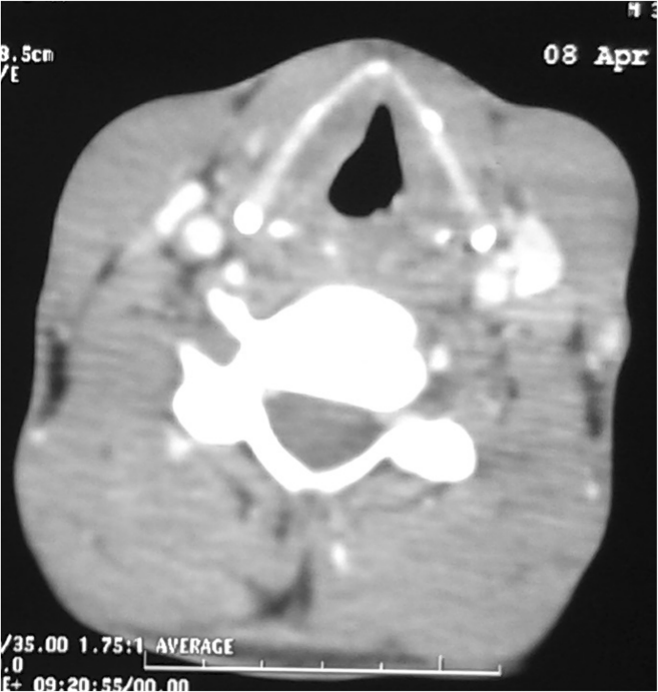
Axial computed tomography section showing thickening of the left vocal cord.
